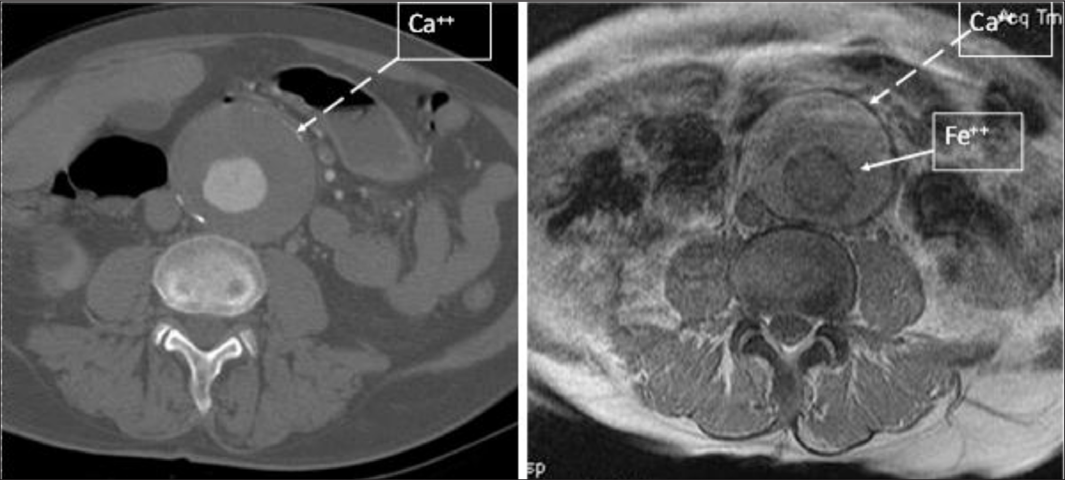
Transverse T2*-weighted MRI (right panel) of a large AAA showing abluminal and subadventitial low-signal rings. The corresponding contrast-enhanced CT transverse slice (left panel) shows subadventitial hyperdense calcifications (Ca++) but fails to replicate this finding around the aortic lumen, as CT is less sensitive to low iron (Fe++) concentrations than T2*-weighted MRI.
